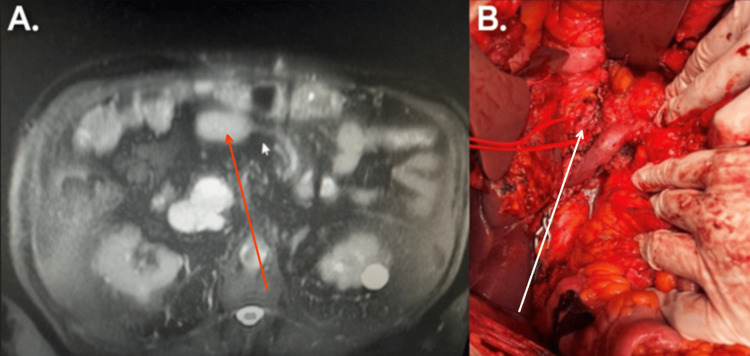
(A) CT scan showing a mucinous pancreatic neoplasm in the head of the pancreas (red arrow). (B) Intraoperative view of total pancreatectomy with right hemicolectomy (white arrow).
